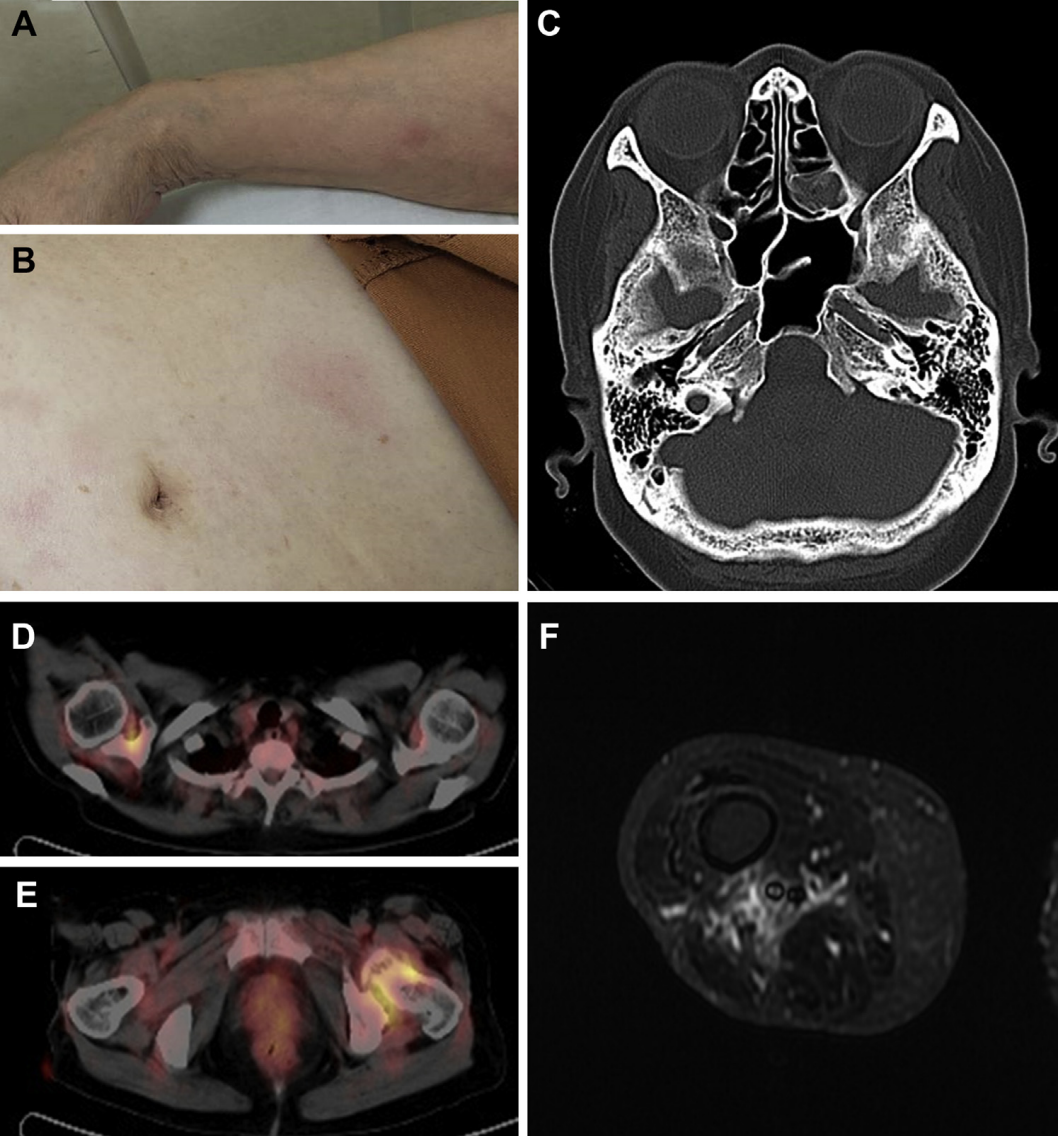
Skin rash and radiographic findings at time of admission. A and B, Erythematous plaques are visible on the upper limb (A) and abdomen (B). C, Sinus computed tomography image showing bilateral ethmoid sinusitis. D and E, Positron emission tomography showing ^18^F-fluorodeoxyglucose accumulation at the right shoulder (D) and left hip joint (E). F, Magnetic resonance imaging of the lower right thigh showing mild edematous changes between the deep muscles.
